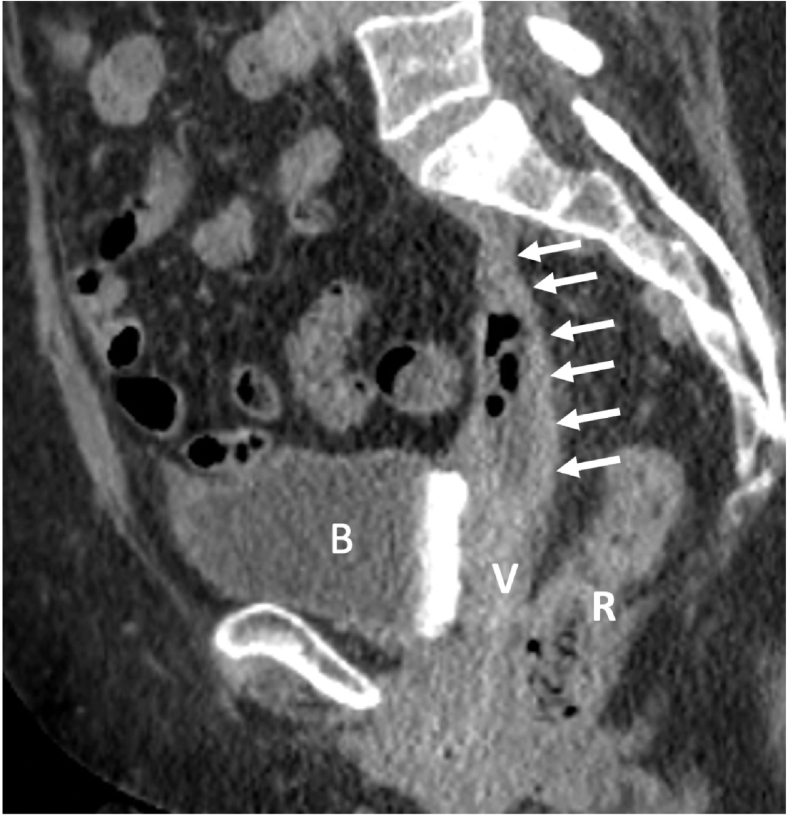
CT abdomen/pelvis sagittal view. CT abdomen/pelvis sagittal view showing infected mesh with fluid and gas collection attached over the sacrum. (B) bladder, (V) vagina, (R) rectum.
